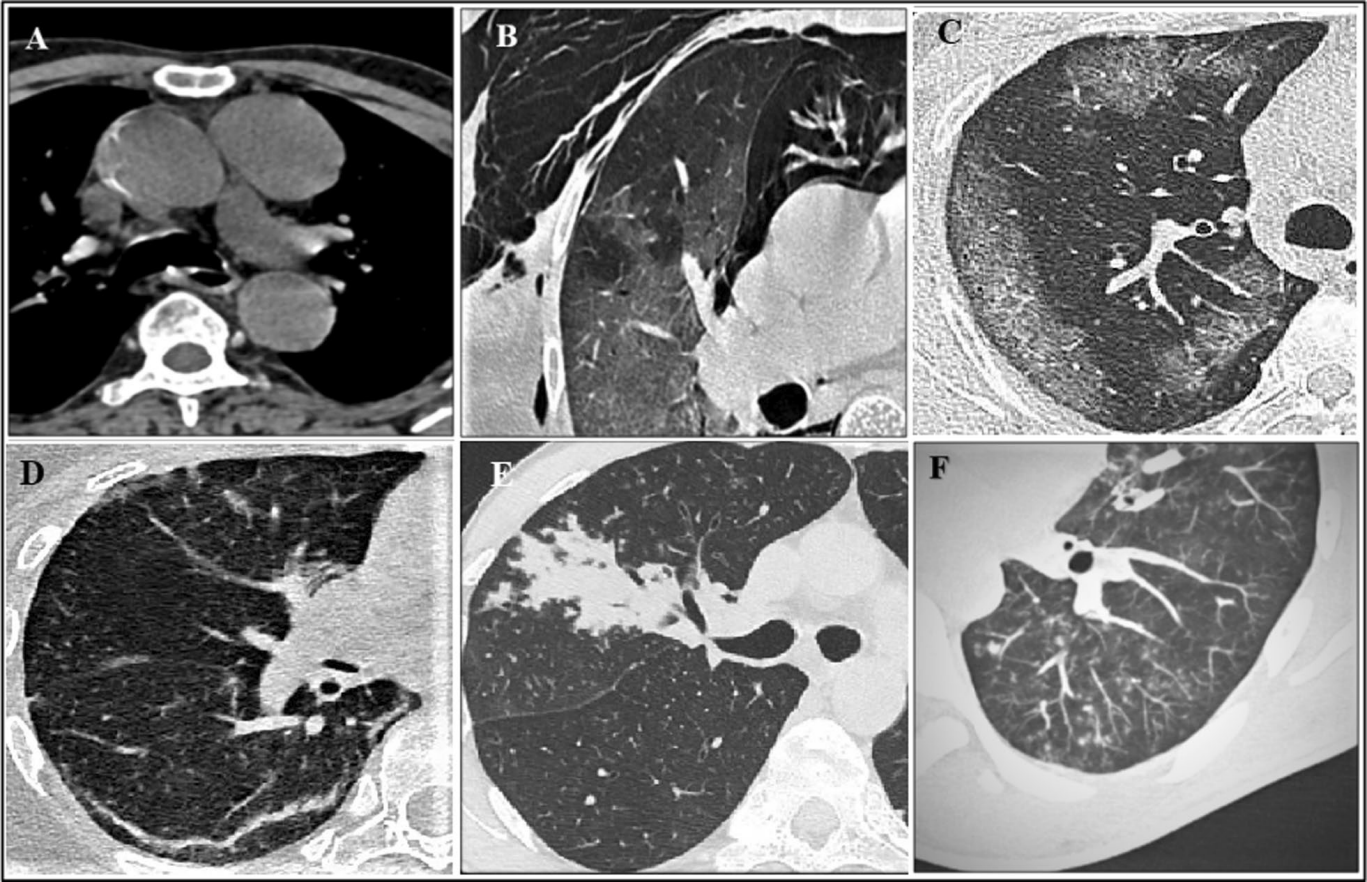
Axial section through HRCT chest of different patients demonstrating atypical imaging features. A Mediastinal lymphadenopathy; B ground-glass opacifications, pneumothorax, pneumomediastinum and subcutaneous emphysema; C peripheral confluent ground-glass opacifications; D subpleural curvilinear opacifications; E isolated segmental consolidation; F tree-in bud nodules
